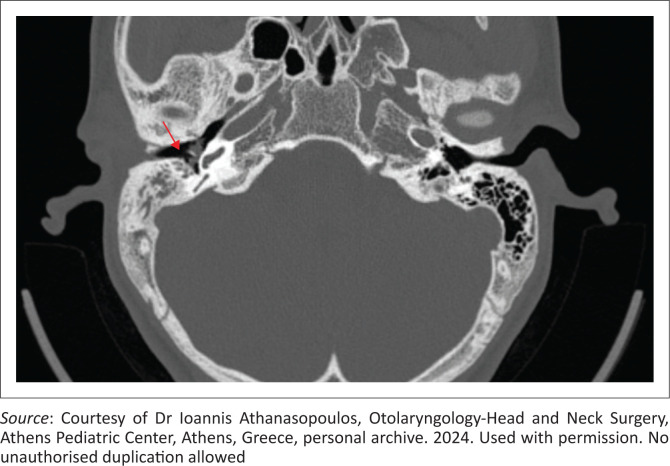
Axial CT image of the temporal bone in a 15-year-old male patient with right-sided cholesteatoma, showing soft tissue opacification and erosive changes of the ossicular chain (long process of the incus, red arrow), contributing to conductive hearing loss.
